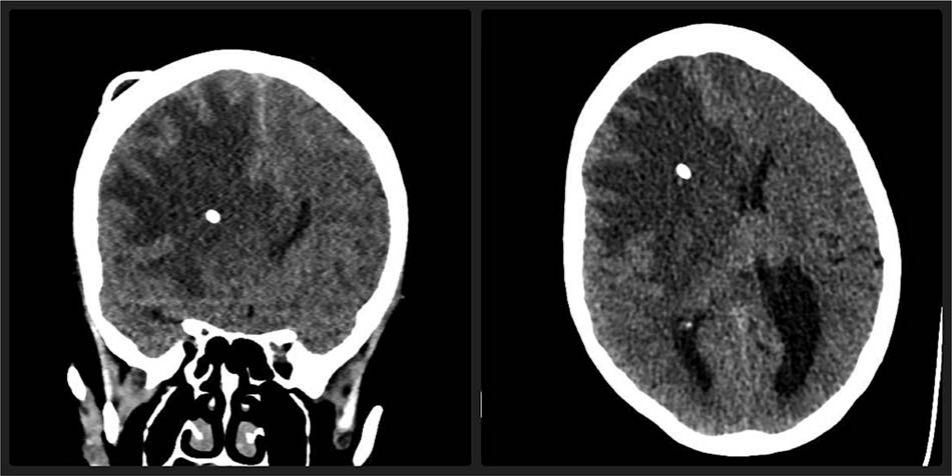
Axial and coronal non-contrast CT scan of the brain demonstrate a marked hypodense area in the right frontal lobe, predominantly involving the white matter, associated with significant mass effect and midline shift. Note the hyperdense catheter inside brain parenchyma.
